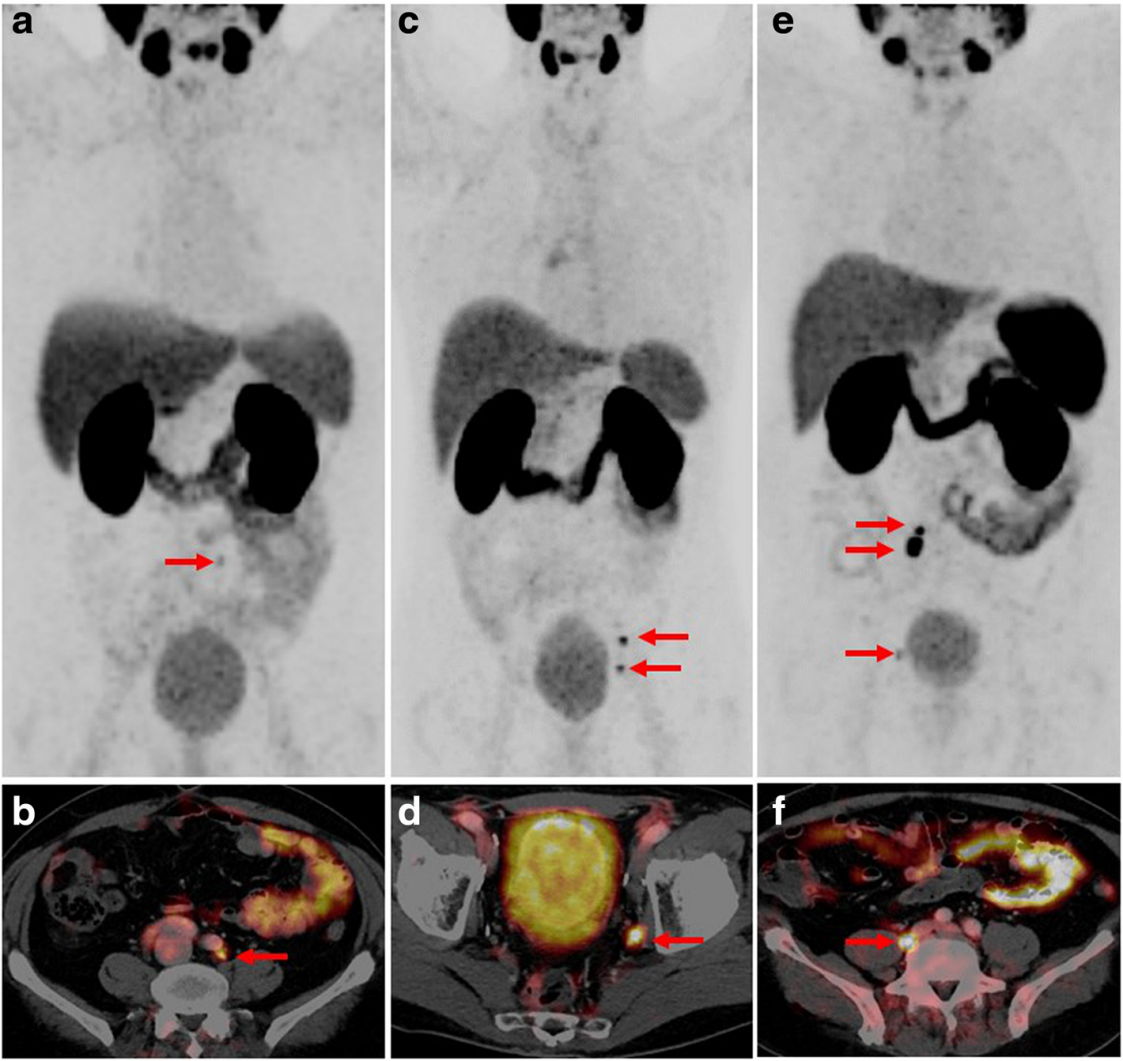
MIP a, c, e and fused ^68^Ga-PSMA ligand PET/CT b, d, f of a patient presenting with low ^68^Ga-PSMA ligand uptake in the left common iliac artery (A and B, SUV mean 3.5), a patient presenting with moderate ^68^Ga-PSMA ligand uptake in the left internal iliac artery (SUV mean 7,8) and in the left external iliac artery (C and D) and a patient presenting with intensive, focal ^68^Ga-PSMA ligand uptake behind the right common iliac vein twice (E and F, SUV mean 17,9), Note the low uptake adjacent to the urinary bladder on the right side in the MIP images (E)
